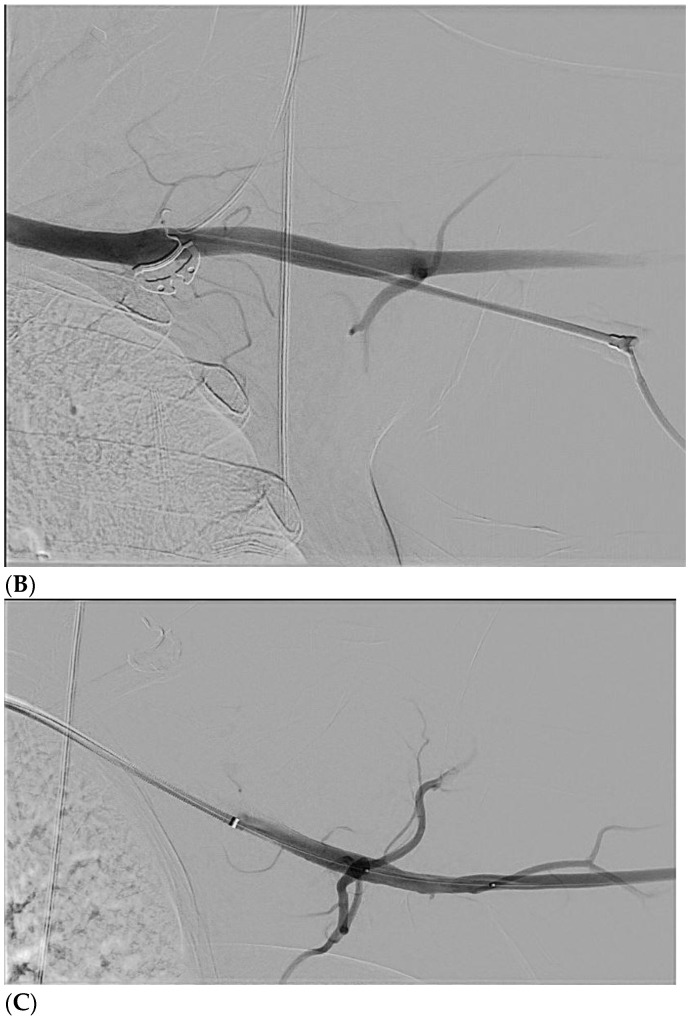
(A): Left axillary region. Puncture was made in the third segment of the AxA. The picture shows a 12F sheath (yellow star), which was introduced into the AxA. In addition, the two preloaded Perclose ProGlide PVCDs (Abbott Vascular, Santa Clara, CA, USA) (yellow arrows) can be seen. (B): Baseline angiography of the axillary artery (AxA) after ultrasound guided puncture in the third segment of the vessel. Consistent with the results of a previous CT scan, no relevant atherosclerotic changes were detected in this vessel segment, and the vessel diameter was >5 mm. The patient required pre-planned treatment for chronic thoraco-abdominal aortic aneurysm. (C): After successful fenestrated endovascular aortic repair (FEVAR) with fenestrations for the left and right renal artery, coeliac trunc, and superior mesenteric artery, closure of the axillary puncture site was performed using two Perclose ProGlide PVCDs (Abbott Vascular, Santa Clara, CA, USA). The control angiogram via the left femoral access showed no complications at the AxA puncture site.
